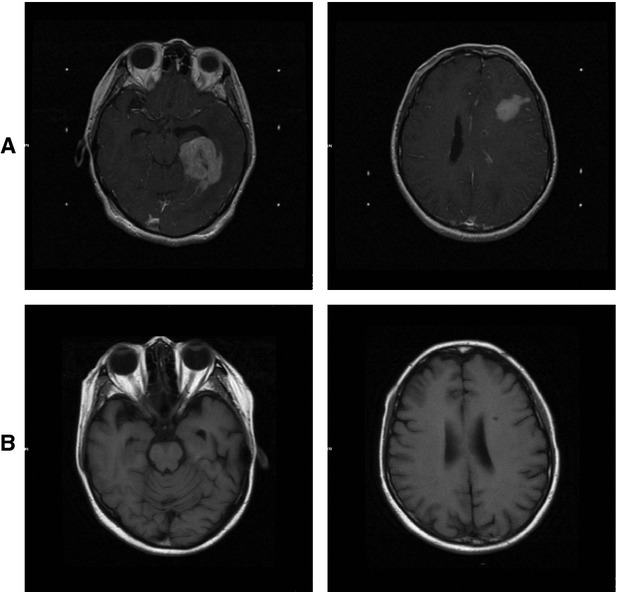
In Patient 2, MR scan shows multiple mass lesions on bilateral lobi temporalis and the left frontal lobe (A). After two cycles of chemotherapy, MR images show the lesion disappeared (B).
